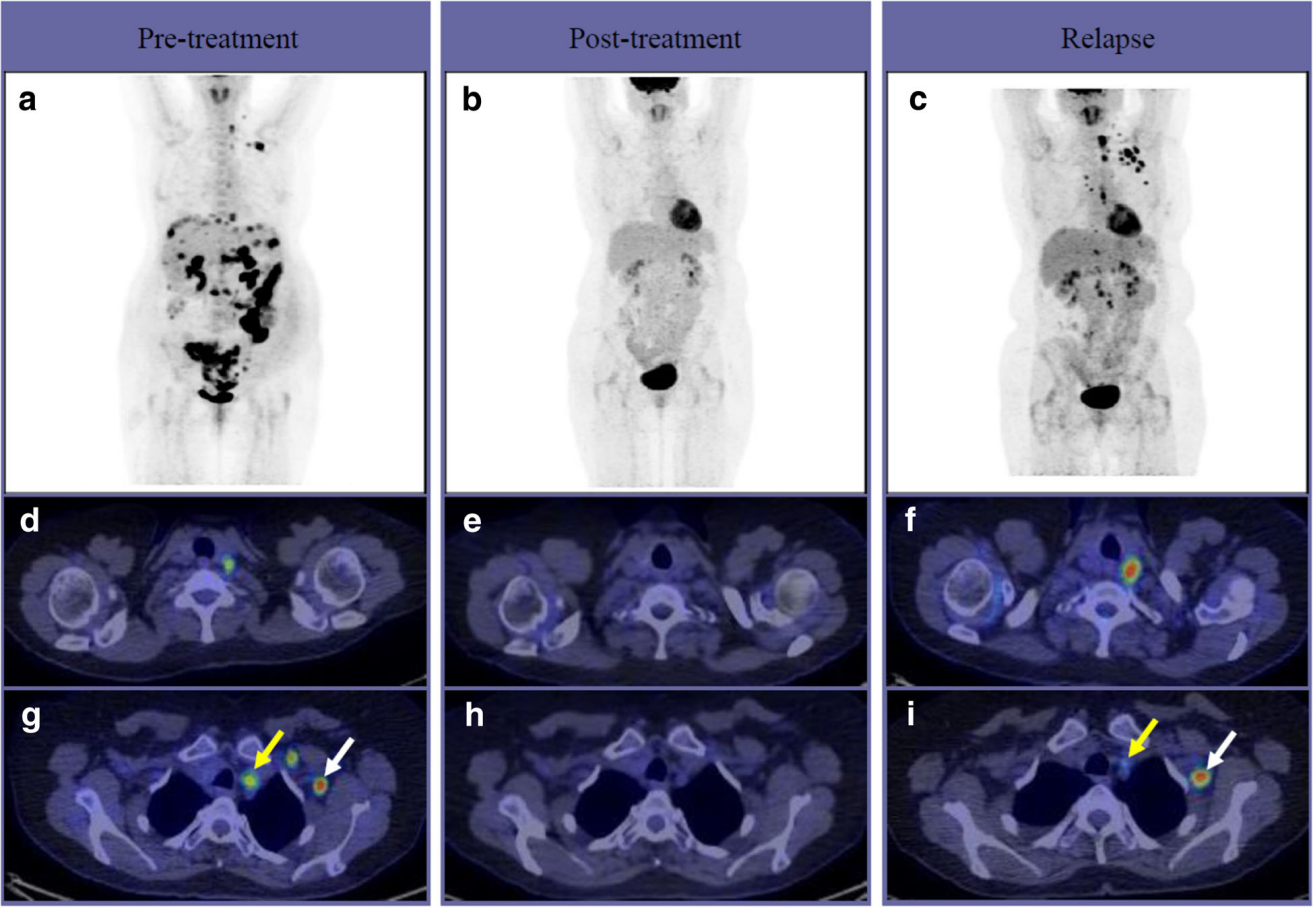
The preoperative whole body PET/CT maximum intensity projection view of the patient with FIGO stage IIIC high grade EOC show, in addition to the abdominal involvement, an extensive FDG uptake in cardiophrenic, parasternal, mediastinal, subclavicular, neck and axillary lymph nodes (a). After 3 cycles of NACT, an interval debulking surgery (with no gross residual disease) and 3 cycles of standard adjuvant taxane-platinum based chemotherapy the FDG uptake has been normalized (b). PET/CT performed 11 months after the end of primary treatment shows metabolic reactivation of paraaortal and supradiaphragmatic lymph nodes as well as new metabolically active supradiaphragmatic lymph nodes (c). Transaxial PET/CT fusion images show the preoperatively detected FDG-avid lymph node profile during treatment and relapse in the neck (d,e,f) as well as in the axillary (white arrow) and mediastinal (yellow arrow) (g,h,i) regions
